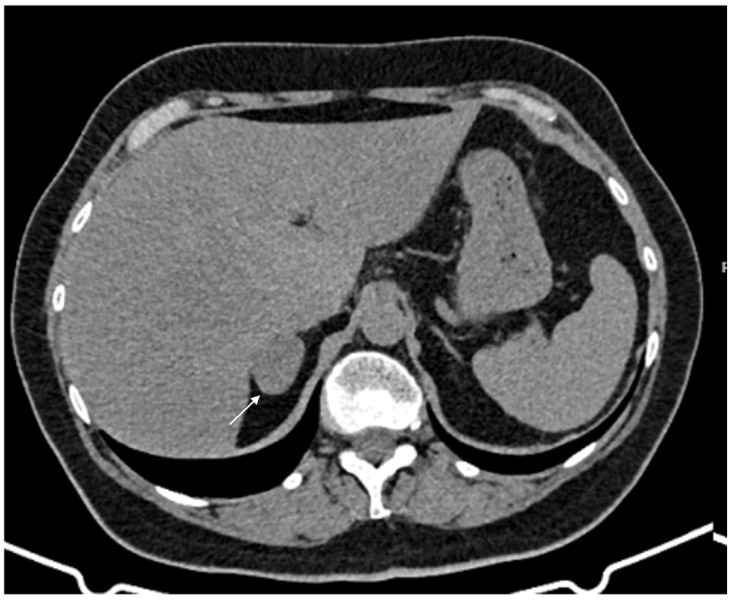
60-year-old woman with adrenal adenoma. Accidental CT finding of a right adrenal mass (arrow) of approximately 3 cm, homogeneous with clear margins and density values lower than 10 HU.
